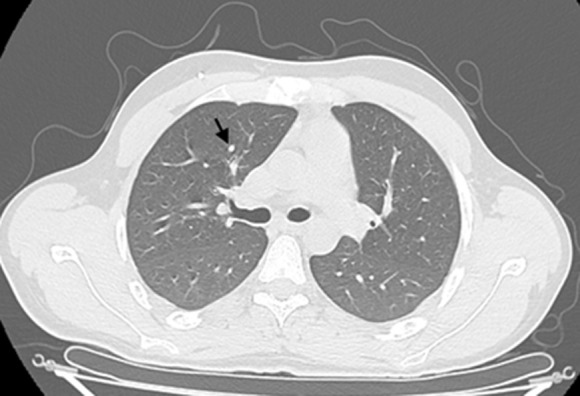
chest computed tomography image showing a non-specific pulmonary micronodule: parenchymal window chest computed tomography image demonstrating a small non-specific pulmonary micronodule (arrow) without associated consolidation or mass effect
